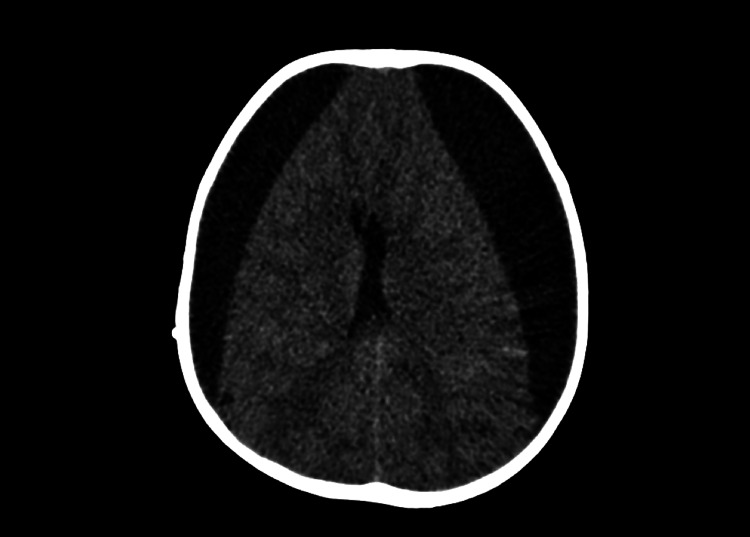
Image of bilateral subdural hygromas on head CT scan
